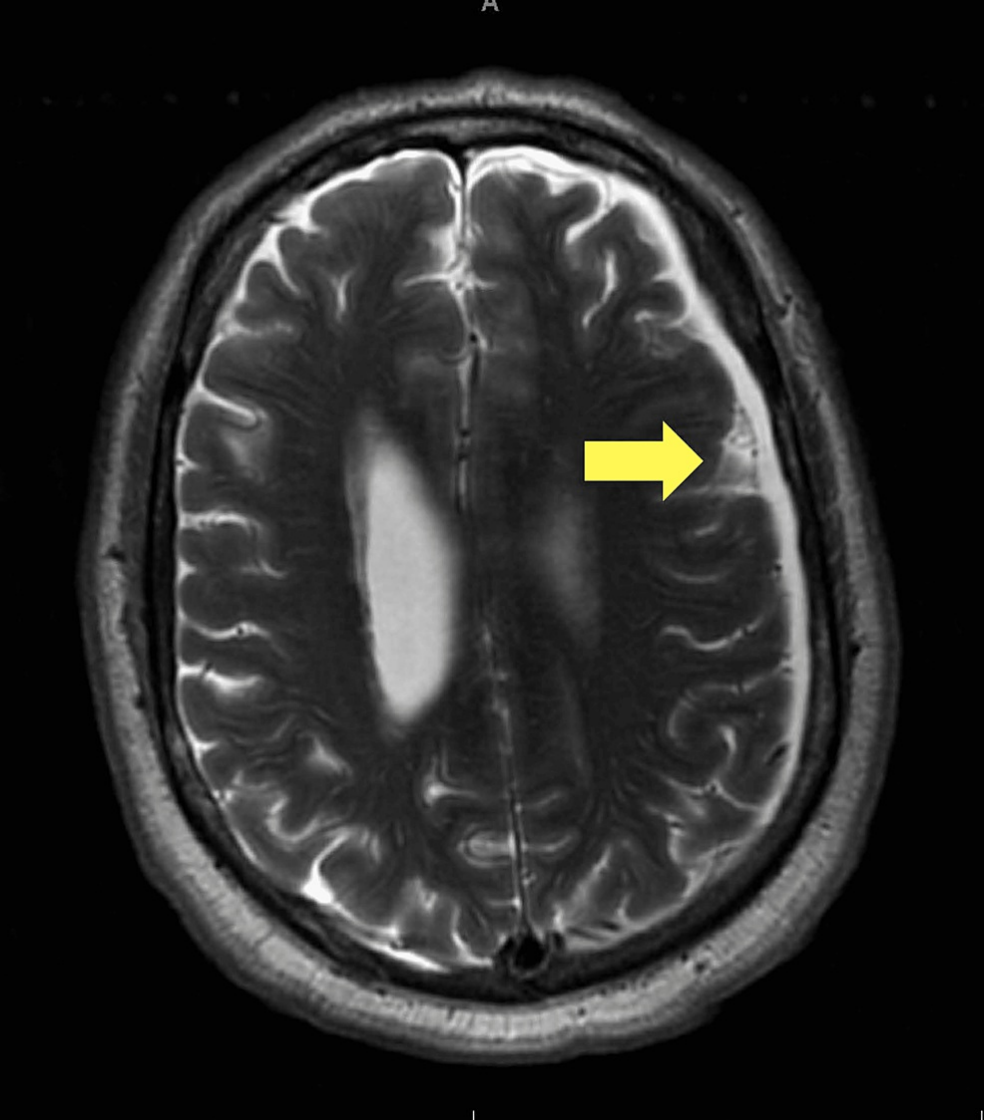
MRI with and without contrast showed subdural fluid collection over the left cerebral convexity suggestive of subacute/chronic subdural hemorrhage versus infectious process.
